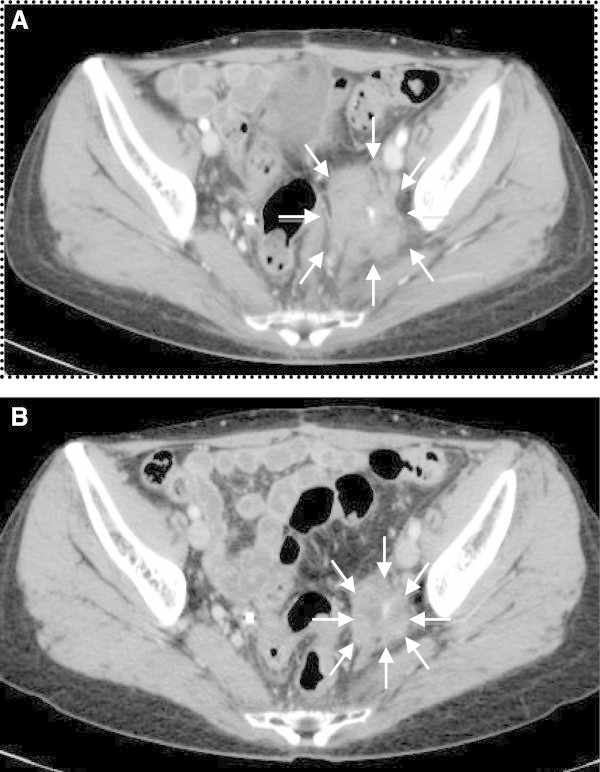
CT images during dienogest therapy. Based on the RECIST criteria, a partial response was obtained at 6 months after initiating oral DNG treatment (A: 0 months; B: 6 months) and the tumor was stable up to 21 months.
